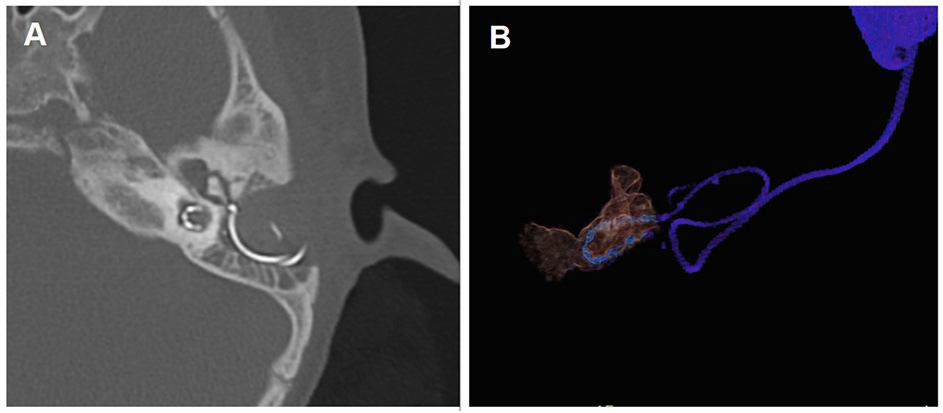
Post-operative computed tomography (CT) scan showing electrodes in CCD patients. (A) CT scan of the temporal bone with electrode in the axial position; (B) Three-dimensional reconstruction of the electrode and common cavity.
